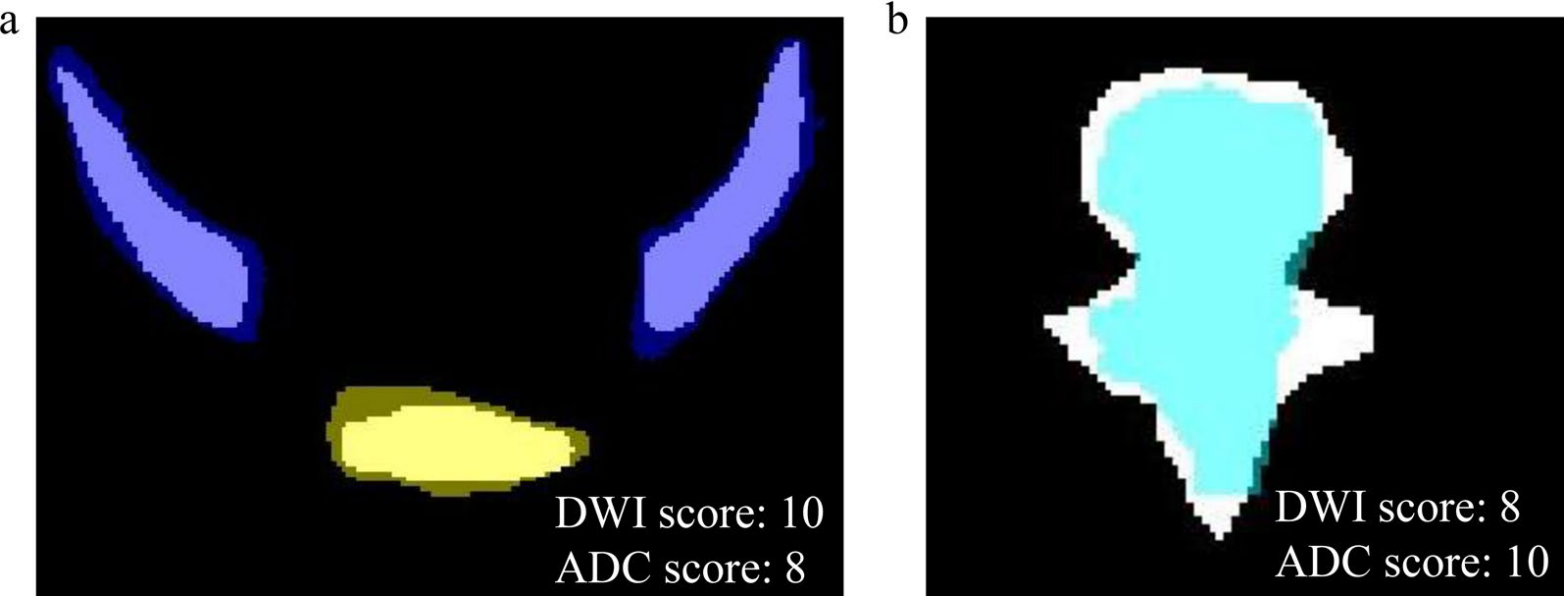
SCORE results on DWI and ADC images. a The range of the CNN-predicted segmentations of sacrococcyx (yellow label) and ilium (deep blue label) was slightly more extensive than the manual segmentations (white background), attaining DSC values of 0.92 and 0.88, respectively. According to the SCORE system, 10 on the DWI image and 8 on the ADC image were obtained. b The range of the CNN-predicted segmentation of lumbar vertebra (light blue label) was slightly smaller than manual segmentation (white background) with a DSC value of 0.82. According to the SCORE system, 8 on the DWI image and 10 on the ADC image were obtained
